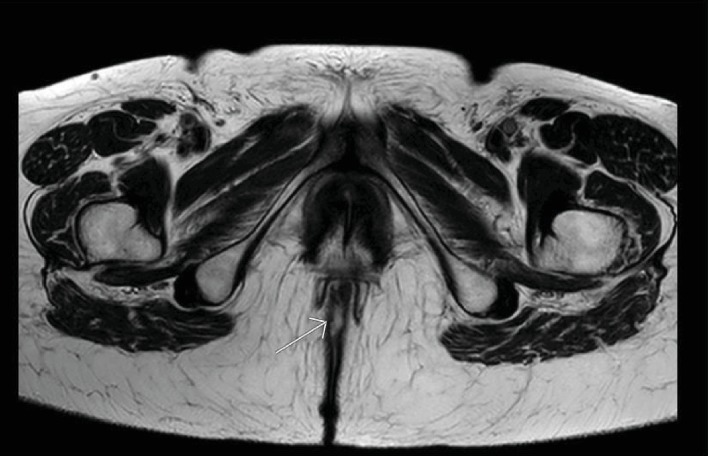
Pelvic MRI (T2W-TSE) showing invasion of the skin but not of the anal sphincters.
